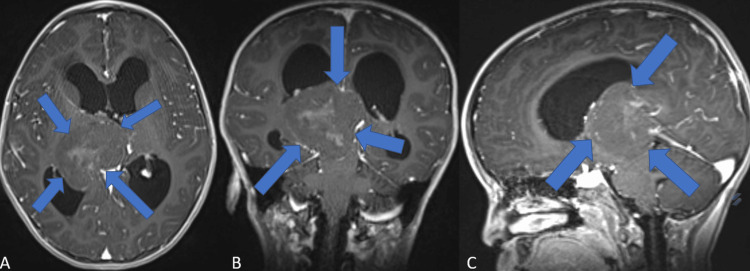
Preoperative contrast-enhanced cranial MRI of a supratentorial ependymoma. A: Axial T1-weighted image with contrast material enhancement showing an isointense pineal lesion displaying intralesional calcified hemorrhagic hyperintense signals, compressing both the lateral and third ventricles. Blue arrows indicate the borders of the lesion. B: Coronal T1-weighted image with contrast material enhancement showing the isointense pineal lesion displaying intralesional calcified/hemorrhagic hyperintense signals, compressing the adjacent structures. Blue arrows are describing the borders of the lesion. C: Sagittal T1-weighted image showing an isointense pineal mass displaying intralesional hyperintensities, compressing the surrounding structures and resulting hydrocephalus. Blue arrows indicate the borders of the lesion.
